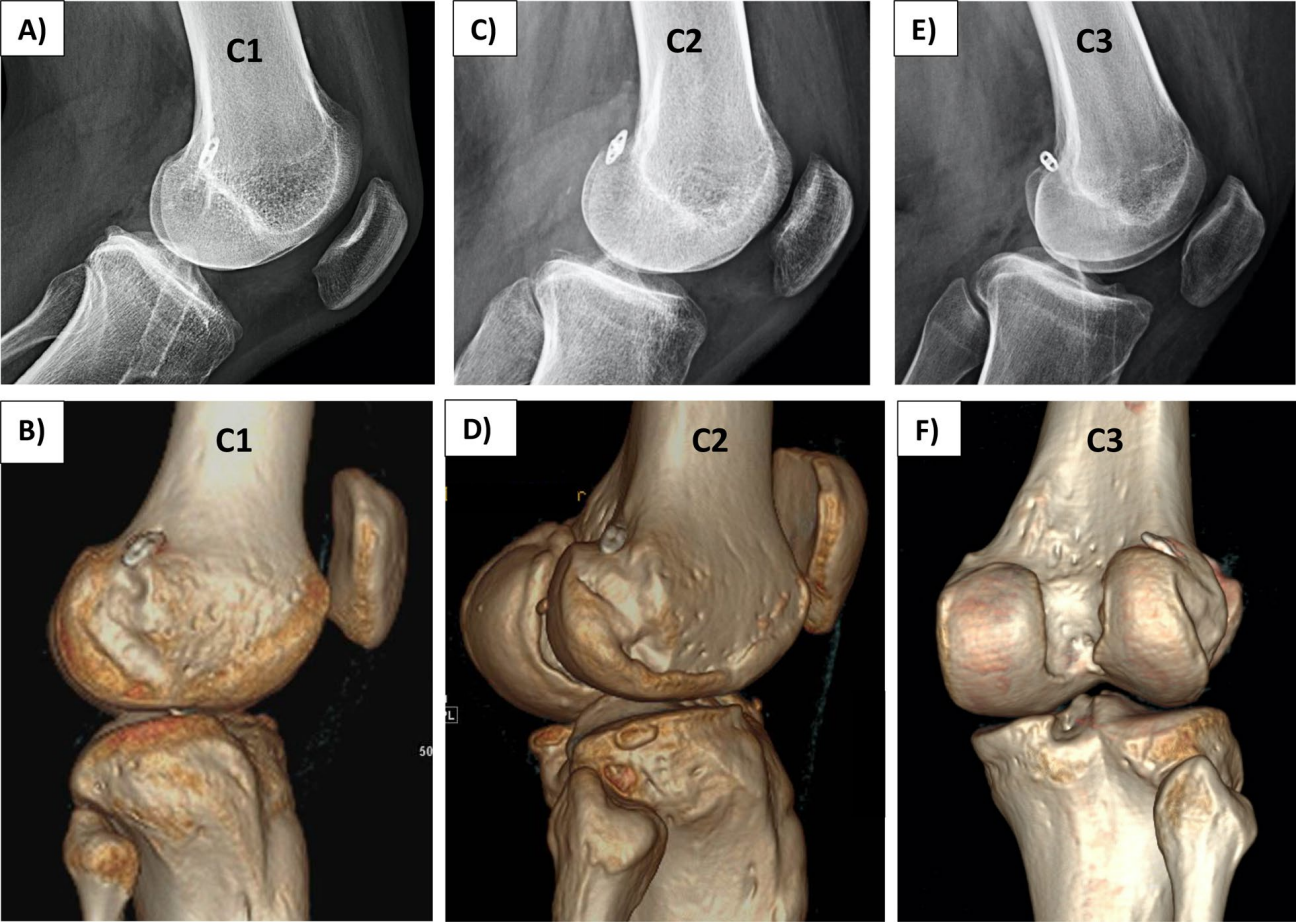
Representative image (X-ray and CT) of femoral tunnel exit type C. Zone C was subdivided into three subzones based on tunnel exist location: 1-lateral (A and B), 2-posterolateral (C and D), and 3-posterior (E and F)
